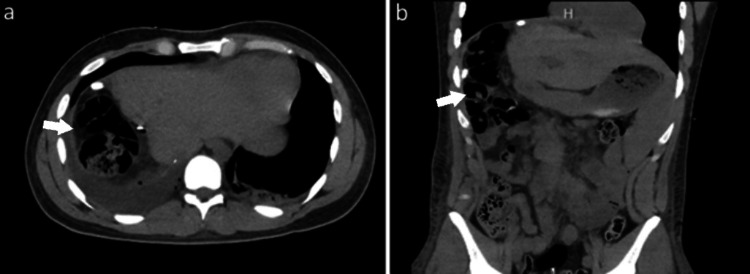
Post-operative images a) Contrast CT - transverse, b) Noncontrast CT - coronal. Arrows represent the site of operation for right hepatectomy.
